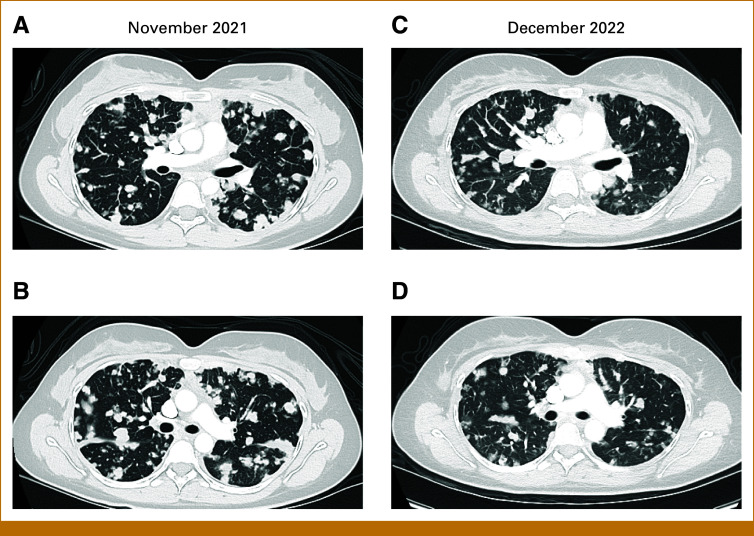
Chest CT showing pulmonary tumor burden (A and B) before treatment with AXL-CAB-ADC ( November 2021) compared with (C and D) post-treatment (December 2022). Despite progression documented as over 20% growth from nadir, imaging reveals overall improvement in ACC pulmonary disease. ACC, adenoid cystic carcinoma; CT, computed tomography.
